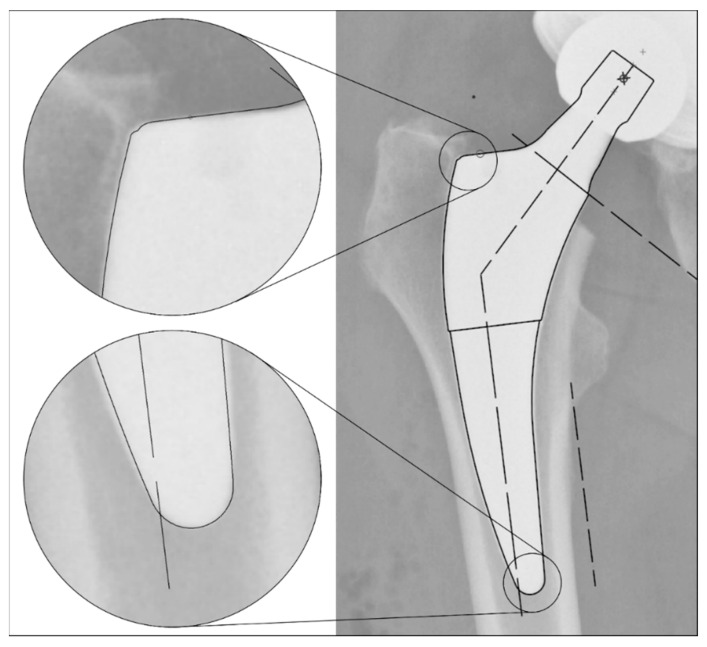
Method for calibrating the X-ray images: Equating the maximum length of the stem (from the shoulder to the tip) in the X-ray image to the template size known from the surgical report. This enables a comparable distance measurement even with different X-ray projections due to different rotation and/or varus/valgus position of the leg.
